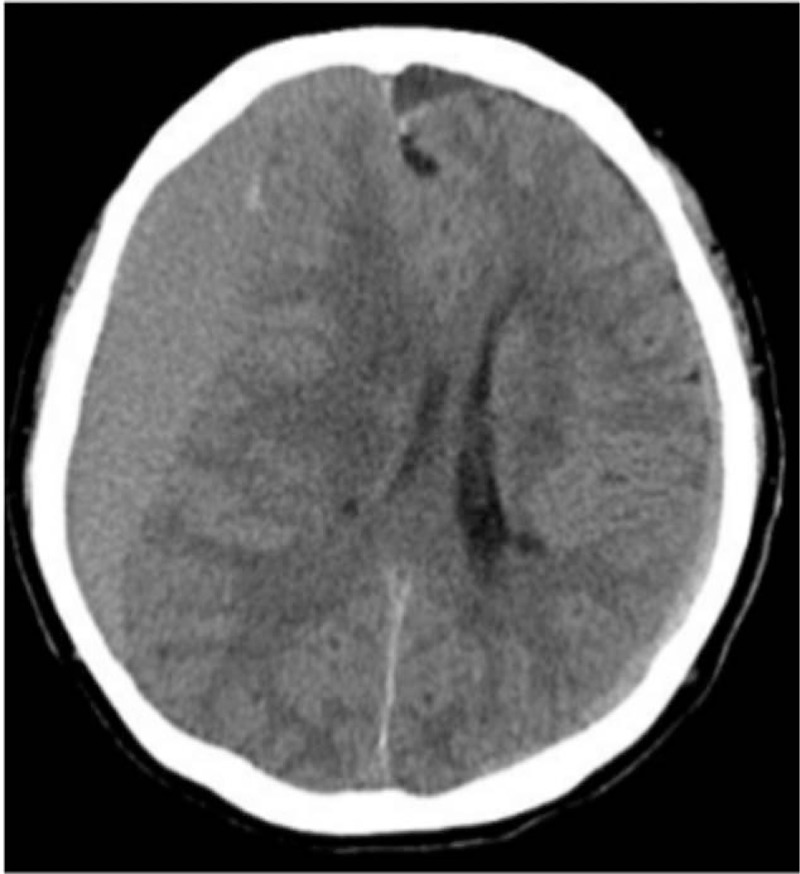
Noncontrast computed tomography after tinnitus and optical illusions shows a hematoma.
